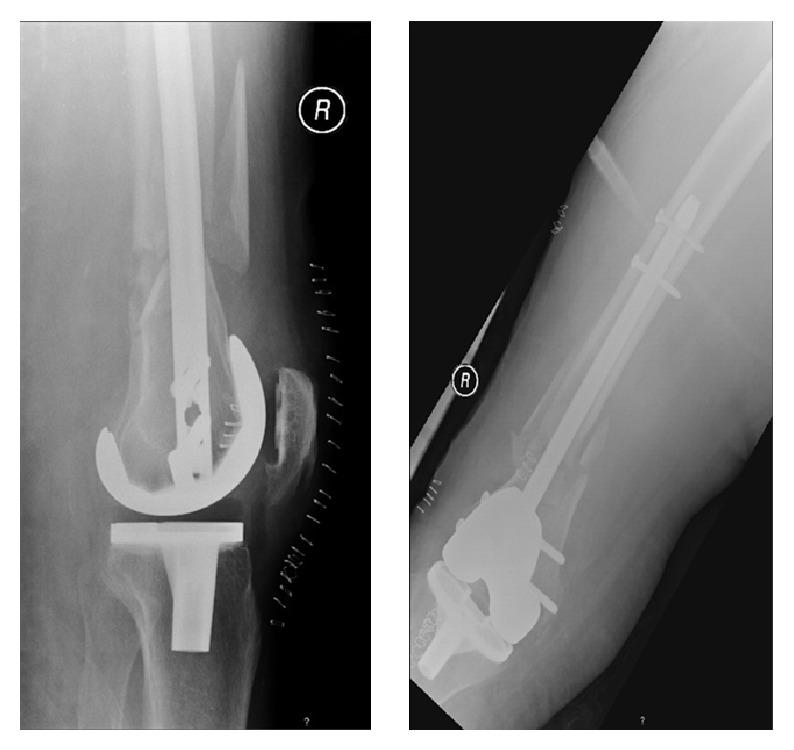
Immediate postoperative AP and lateral X-rays showing a right periprosthetic knee fracture, stabilized with retrograde intramedullary nailing technique.
